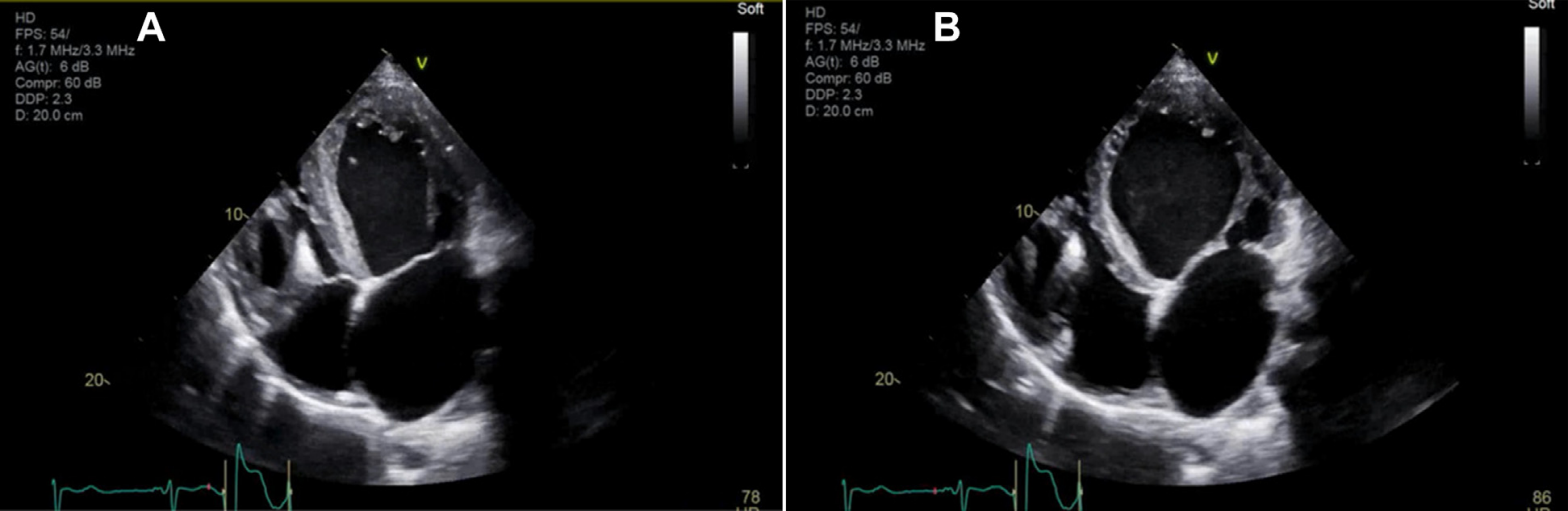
Transthoracic Echocardiogram (A) 4-chamber view in systole reveals a left ventricular ejection fraction biplane of 26% and a hypertrabeculated left ventricle with severe biventricular and left atrial dilatation. (B) 4-chamber view in diastole reveals a left ventricular ejection fraction biplane of 26% and a hypertrabeculated left ventricle with severe biventricular and left atrial dilatation.
